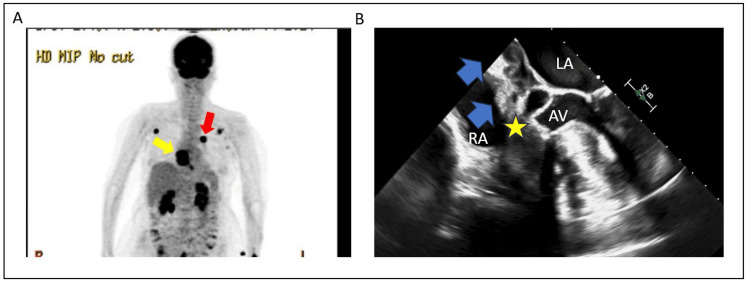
Advanced cardiac imaging A – PET/CT whole body scan showing enhancement of suspected primary lesion on the patient’s back (red arrow) and in the heart at the level of the atria (yellow arrow). B – Transesophageal echocardiogram showing extension of tumor across the inter-atrial septum (blue arrows) with encroachment of the aortic valve. The yellow star denotes the approximate anatomical location of the compact AV node. PET/CT = positron emission tomography/computed tomography; AV = aortic valve; all other abbreviations as per Figure 2.
